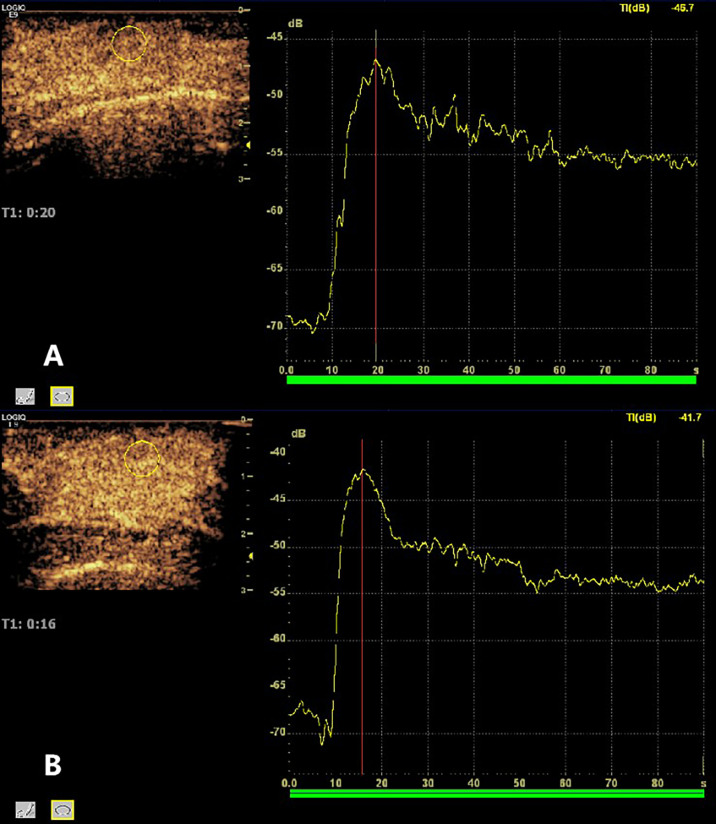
Changes in the CEUS TIC curve before and after ultrasonic therapy. (A) Before ultrasound treatment, peak intensity = -45.7dB, mean transit time = 74.00 s, area under curve =1560.317; (B) After ultrasound treatment, peak intensity = -41.7dB, mean transit time = 85.60s, and area under the curve = 1746.542.
